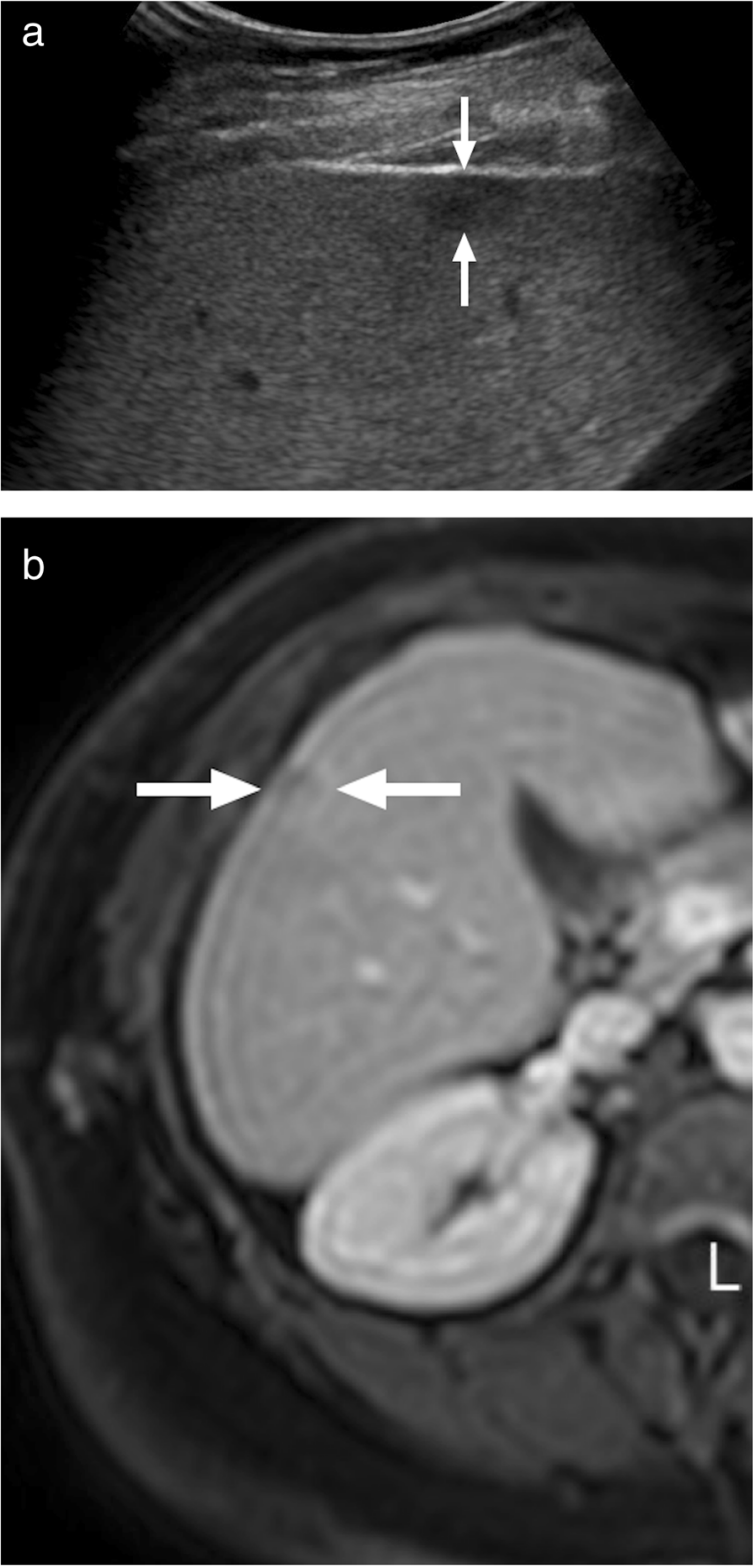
A 50-year-old female with known breast cancer in remission was referred to MRI study after detection of a subcentimeter focal liver lesion on US exam. a US study showed subcapsular, hypoechoic solid appearing mass (arrows). b Axial plane postcontrast T1W image showed the same lesion as a hypointense mass with peripheral contrast enhancement (arrows). DW images and ADC map confirmed significant diffusion restriction (not shown). Percutaneous biopsy confirmed eosinophilic liver abscess with no evidence of metastatic disease. Subsequent peripheral blood smear also confirmed hypereosinophilia
